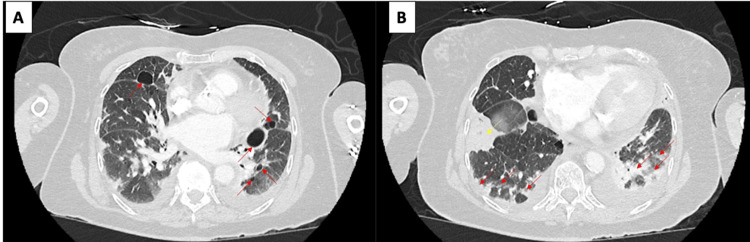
CT of the chest. (A) Bilateral thin-walled pulmonary cysts. (B) Bibasilar fibrotic changes with patchy consolidation and atelectasis Red arrowheads indicate bibasilar fibrotic changes with patchy consolidation and atelectasis; the yellow arrowhead identifies the medial subsegmental right middle lobe pulmonary arteriovenous malformation CT: computed tomography
